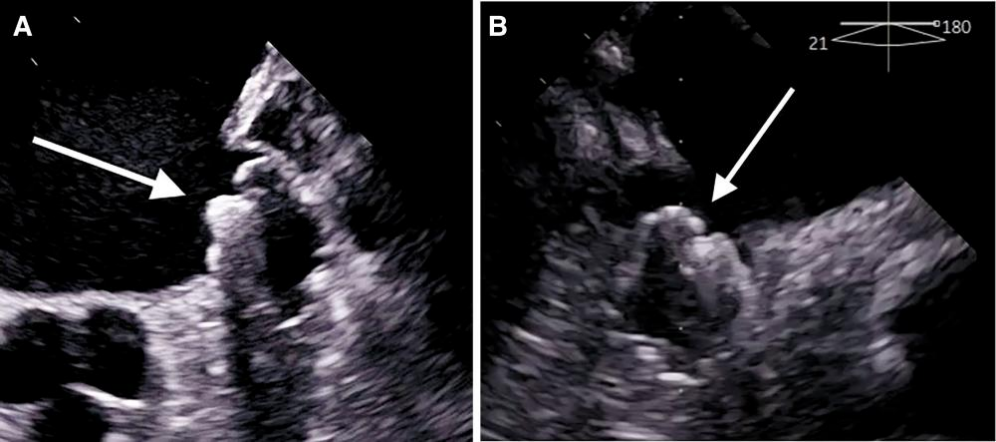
Transoesophageal echocardiography depicting left atrial appendage closure after implantation of the WATCHMAN FLX device (arrows).
